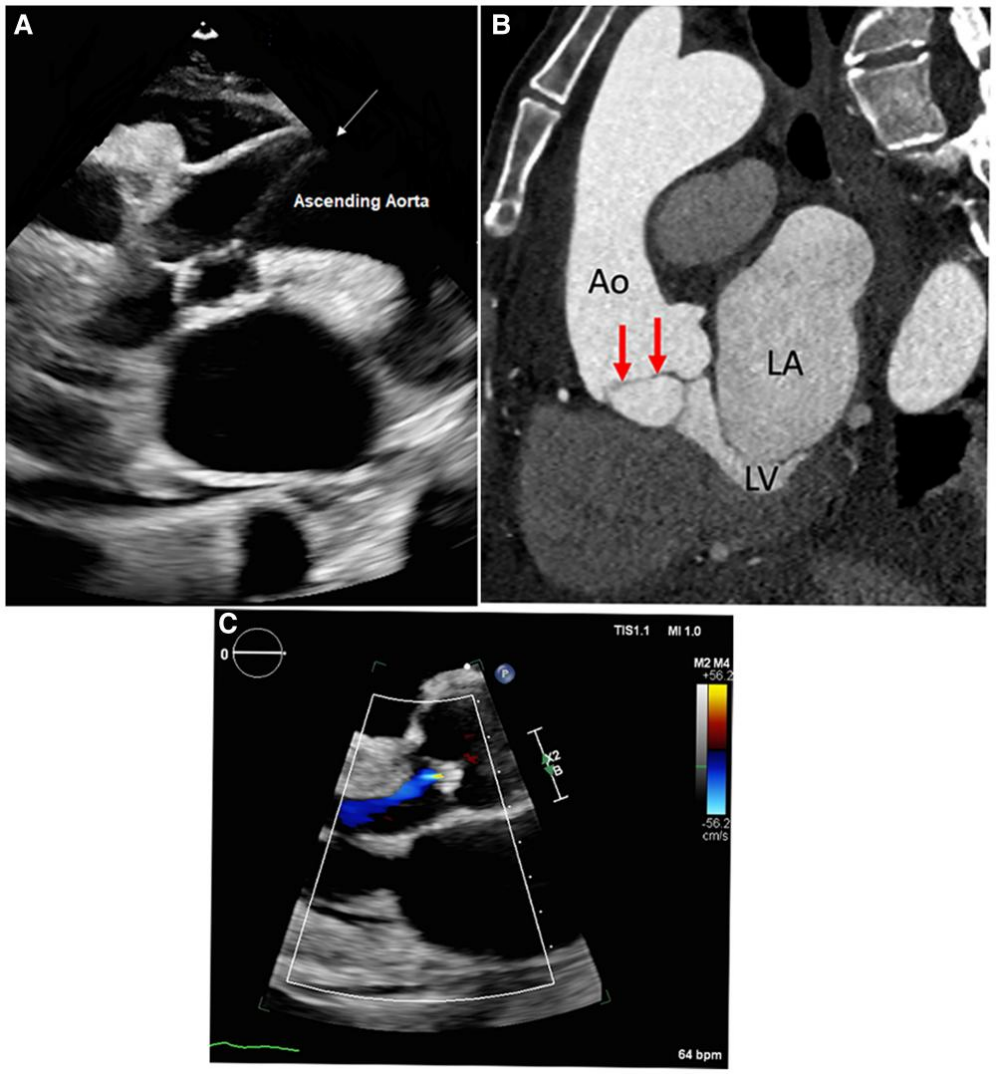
(*A*) Transthoracic echocardiogram with concern for linear echoes within the aorta suggestive of dissection (white arrow). (*B*) Sagittal oblique reformatted multiplanar image from a retrospectively gated cardiac computed tomography angiogram: There is a thin linear filling defect (inferior to Ao) along the right aortic cusp seen intermittently during the cardiac cycle without any associated aortic wall haematoma or irregularity. This corresponds to the abnormality seen on echo. (*C*) Mild aortic regurgitation demonstrated on transthoracic echocardiography. This is the most common finding associated with fibrous band. Ao, aorta; LA, left atrium; LV, left ventricle.
